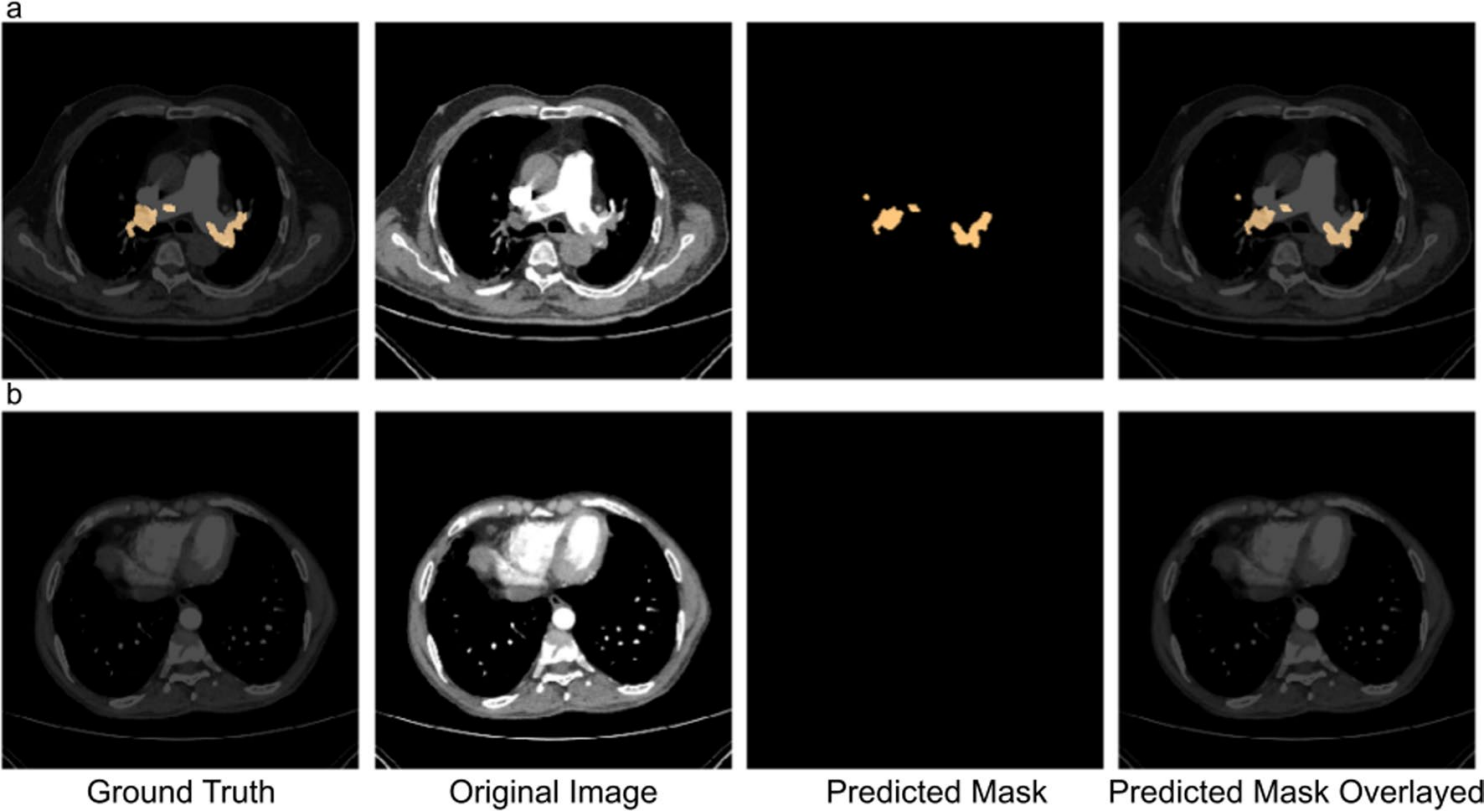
Example of model prediction on a CT slice with a embolism present and b no embolism
